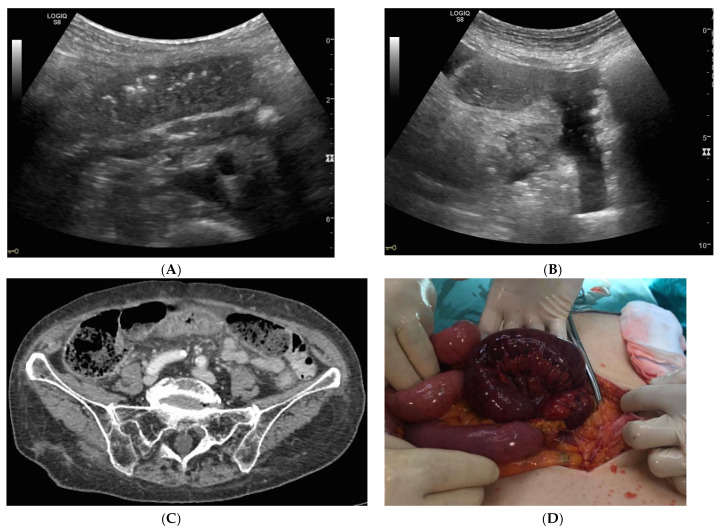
Complicated SBO. (A,B) Bowel loops are dilated, akinetic, with parietal and valvulae conniventes thickening and parietal stratification. There is free fluid between bowel loops. (C) CT with IV contrast axial image. Bowel loop with thickened and stratified wall with hypointense submucosa (image A). In the left quadrant, a dilated bowel loop with a thin layer and feces sign is visible (image B). (D) Laparotomic view of a dilated intestinal loop in necrosis.
